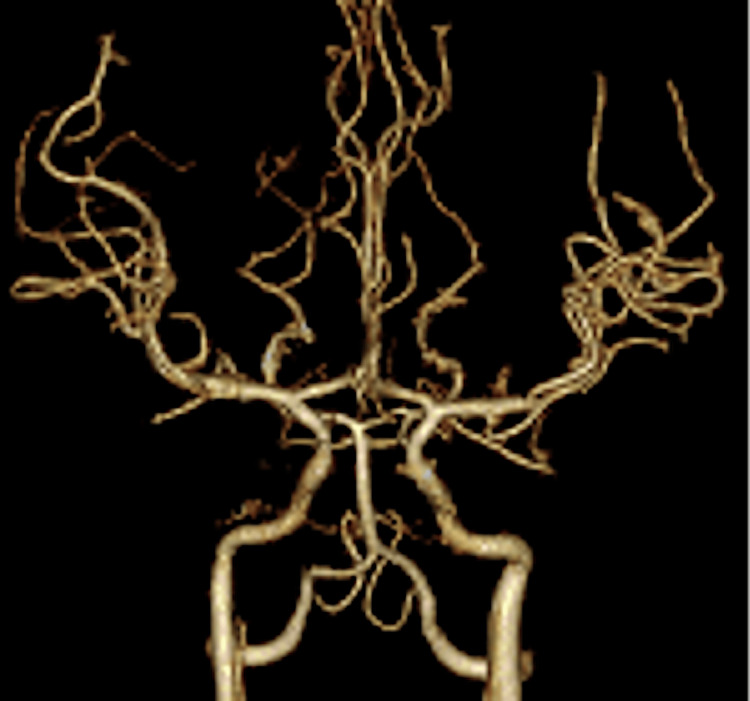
A CT angiography on the second day from the onset did not show any suspicious regions of cerebral vasospasm nor brain edema. CT: computed tomography
